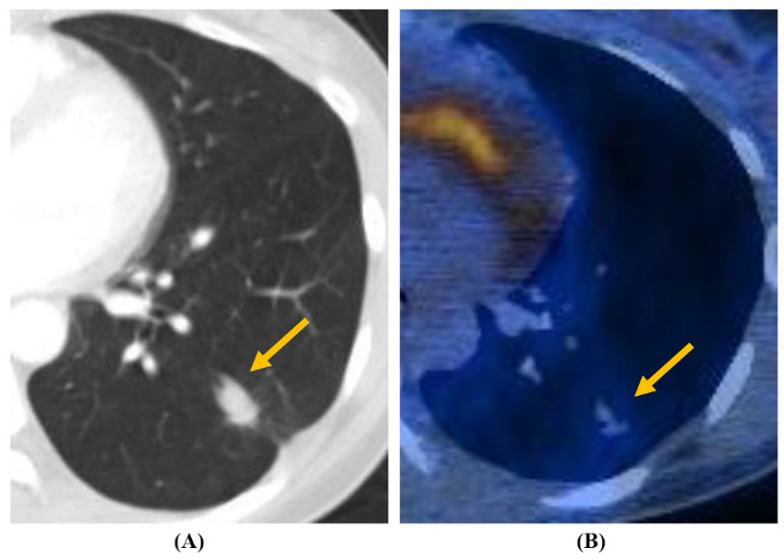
Non-cavitary *Aspergillus* nodule (arrowhead) from a 45-year-old woman. (A) Chest CT showed a 16 mm smooth oval nodule in the peripheral portion of the left lower lobe. (B) PET/CT uptake showed a SUV_max_ value of 1.2.
